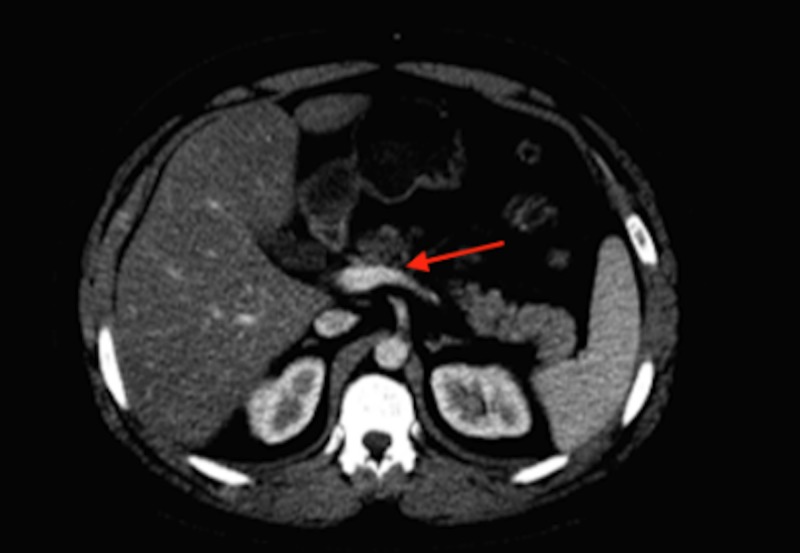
CT abdomen with contrast demonstrating acute pancreatitis
